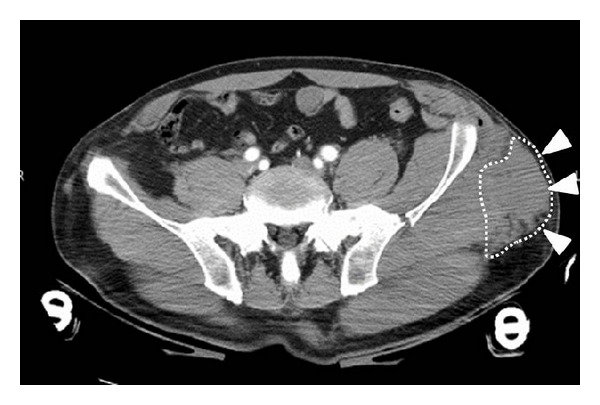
CT axial image around the pelvis. Initial evaluation revealed a narrow low-density area around the left greater trochanter and fracture of the iliac wing.
